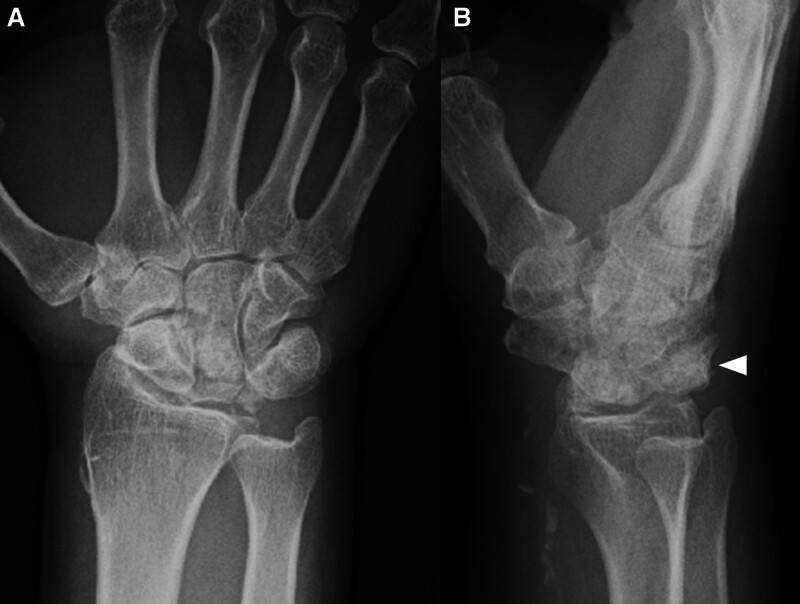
Initial plain radiographs. (A) Anteroposterior and (B) lateral view indicate osteoarthritis of wrist and segmented lunate bone (arrowhead).
